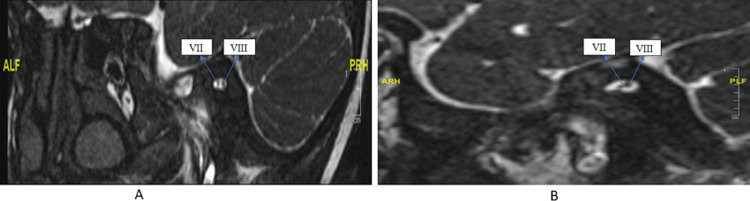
(A) MRI internal auditory canal showed right IAC has two nerves that may represent both the facial nerve (VII) and cochleovestibular nerve (VIII), and (B) MRI internal auditory canal showed left IAC has two nerves that may represent both the facial nerve (VII) and cochleovestibular nerve (VIII). IAC: Internal auditory canal
